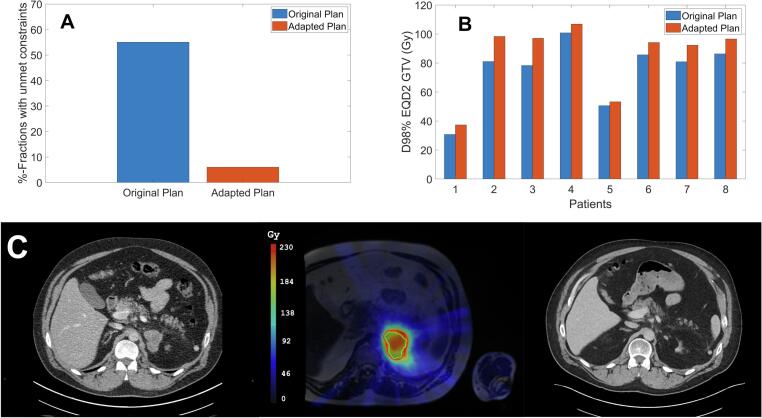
Percentage of fractions exceeding organ-at-risk (OAR) dose constraints for the original treatment plan compared to the online adaptive plan (A). Cumulative EQD2 (Gy) to 98% of the gross tumor volume (GTV) for each patient, comparing the original treatment plan with the online adaptive plan (B). Contrast-enhanced CT imaging prior to the initial treatment course in 2021, revealing a metastasis in the left adrenal gland (C-left); total EQD2 (α/β10 for tumor and α/β3 for OARs) delivered dose distribution following three stereotactic body radiotherapy (SBRT) courses (C-middle); and post-treatment contrast-enhanced CT imaging in 2025 (C-right).
